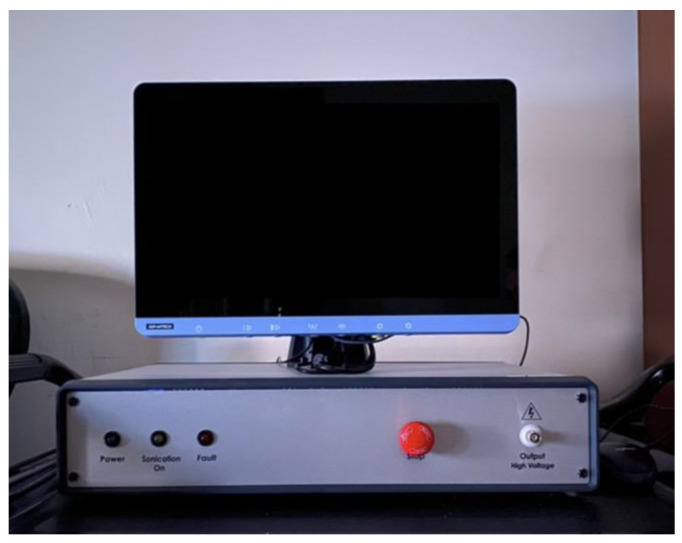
Brainsonix BX Pulsar 1002 ultrasound console.
